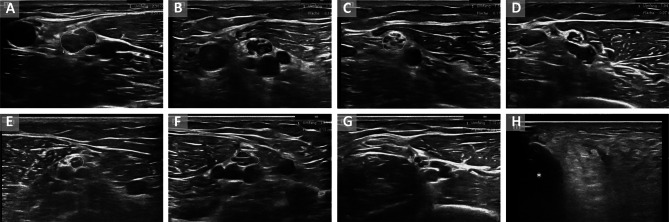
Ultrasound examples. A-G cross-sectional areas (CSA) of median nerves at the upper arm in patients with different hereditary polyneuropathies, A CMT1A, 33 m^2^, B other CMT1/4 (in this example CMT1B), 19 mm^2^, C CMTX, 17 mm^2^, D HNPP, 7 mm^2^, E CMT2F, 8 mm^2^, F ATTRv amyloidosis stage 1, 15 mm^2^, G Fabry’s disease, 8 mm^2^, H example of muscle alteration of tibial anterior muscle in a patient with CMT 1B (variant in *MPZ*), asterix: tibia
